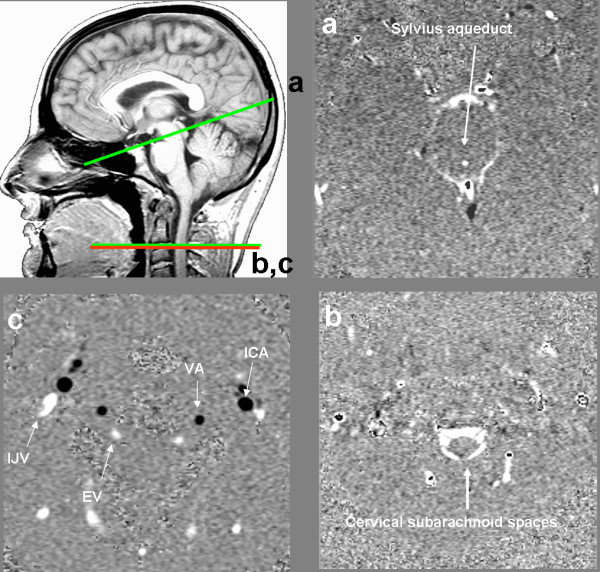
Data acquisition by phase-contrast magnetic resonance imaging. Sagittal scout view sequences were used as localizers to select the anatomical levels for flow quantification. The selected acquisition planes were perpendicular to the presumed flow direction. Sections through the aqueduct of Sylvius (a) and the C2-C3 subarachnoid space (b) were used for CSF flow measurement. By varying the velocity encoding, the same cervical section level (c) was used to measure vascular flows in the left and right internal carotid arteries (ICAs), vertebral arteries (VAs), internal jugular veins (IJVs) and epidural veins (EVs). Figures 1(a), (b) and (c) represent the flow acquisition over the cardiac cycle. See also additional file: Movie1 for the original data used to perform this analysis'.
